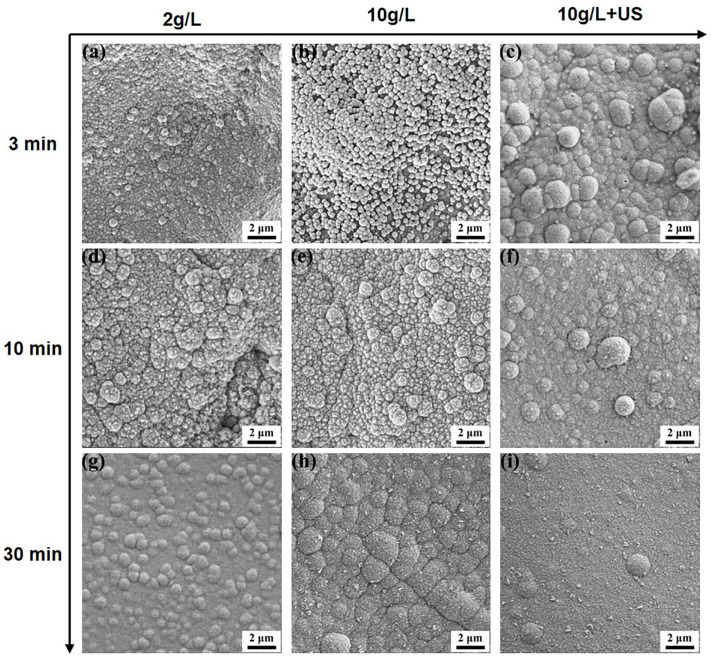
Surface morphology of nickel coatings with different activation methods at different time intervals. (a,d,g) 2 g/L AgNO_3_; (b,e,h) 10 g/L AgNO_3_; (c,f,i) 10 g/L AgNO_3_+ Ultrasonic.
